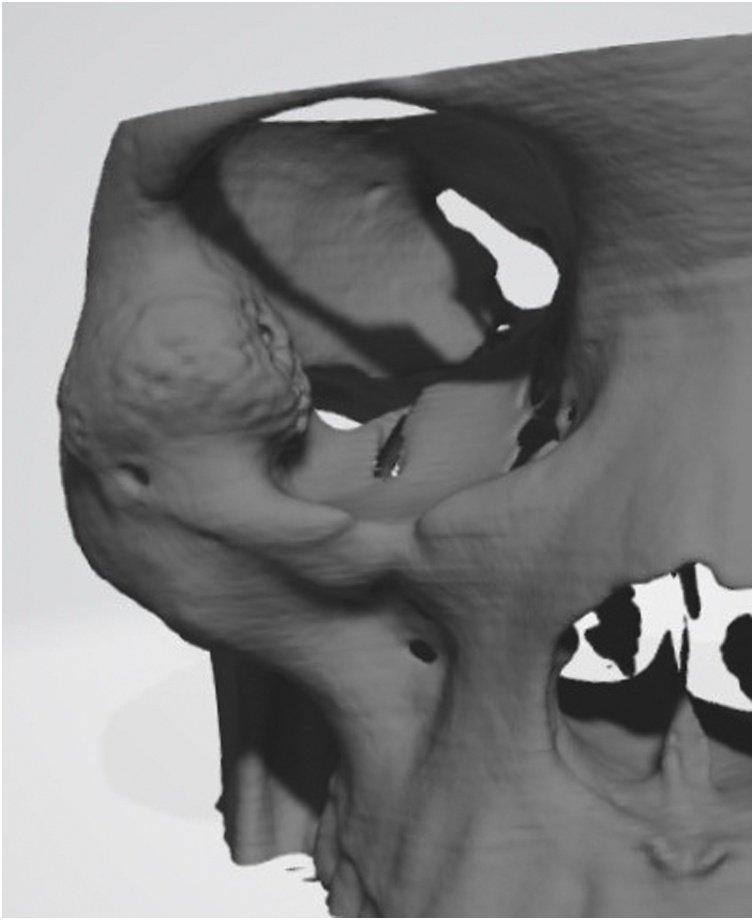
Radiographic picture showing the mass involving the right orbital floor, lateral wall, inferior and lateral rims plus anterior surface of zygomatic body.
